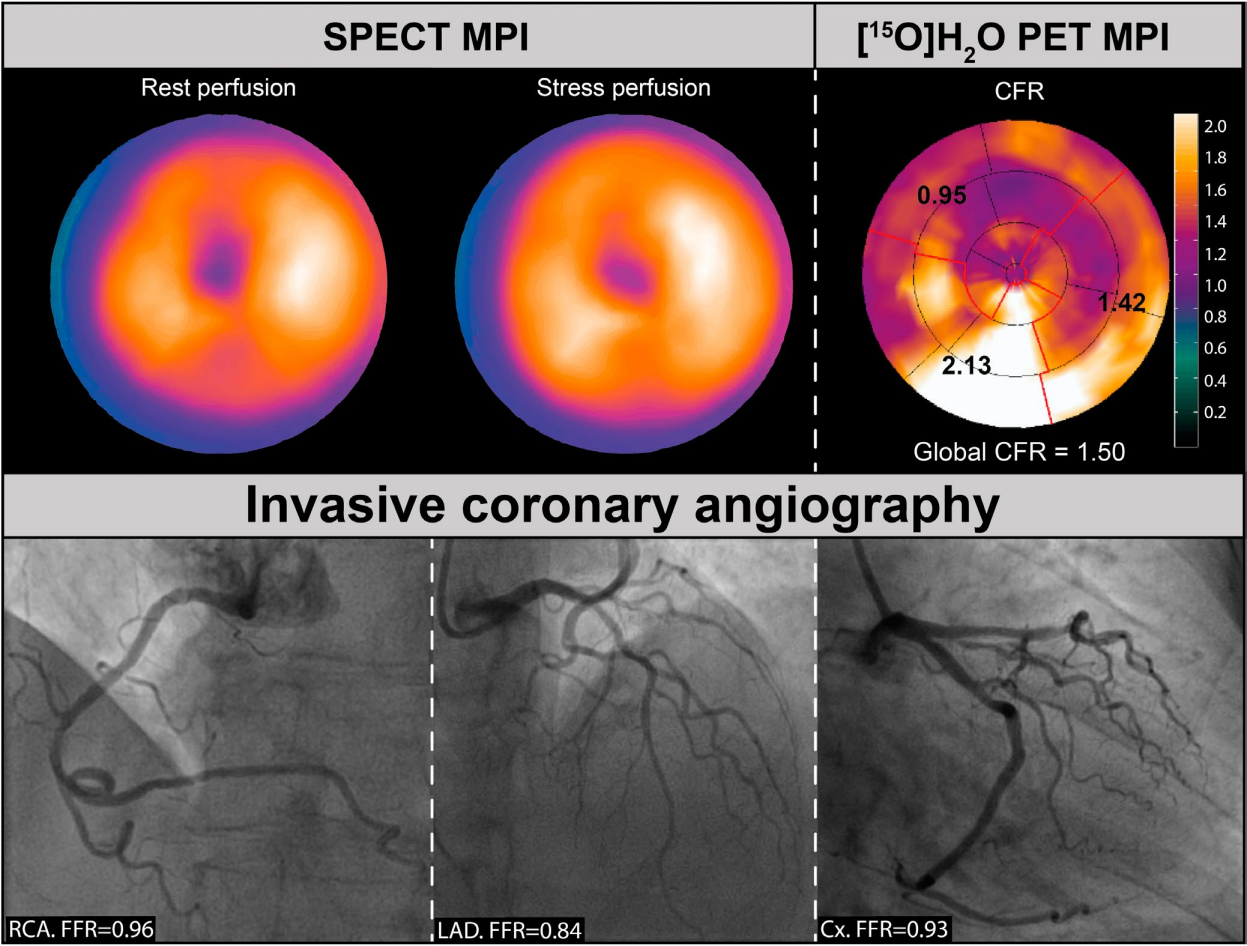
Case example of a negative SPECT and positive [^15^O]H_2_O PET in the absence of obstructive CAD. Case of a patient from the PACIFIC 2 study^25^ with prior PCI of the LAD who underwent SPECT and [^15^O]H_2_O PET MPI because of recurrent angina symptoms. SPECT MPI revealed no ischemia with a summed stress score of 0. Quantification on PET MPI revealed a vascular CFR of 2.13 in RCA territory, .95 in LAD territory and 1.42 in Cx territory, which are indicative of obstructive CAD according to the predefined CFR cutoff of ≤2.5.^85^ However, ICA revealed nonobstructive CAD in all three branches with an FFR of .96 in RCA, .84 in LAD and .93 in Cx. Global CFR of PET MPI is ≤2.0, thereby suggesting that angina symptoms in this female patient with documented CAD could be attributed to CMD, a condition that often coexists with advanced CAD. CAD, coronary artery disease; CMD, coronary microvascular dysfunction; PCI, percutaneous coronary intervention; SPECT, single‐photon emission computed tomography, other abbreviations as in Figures 1 and 2.
